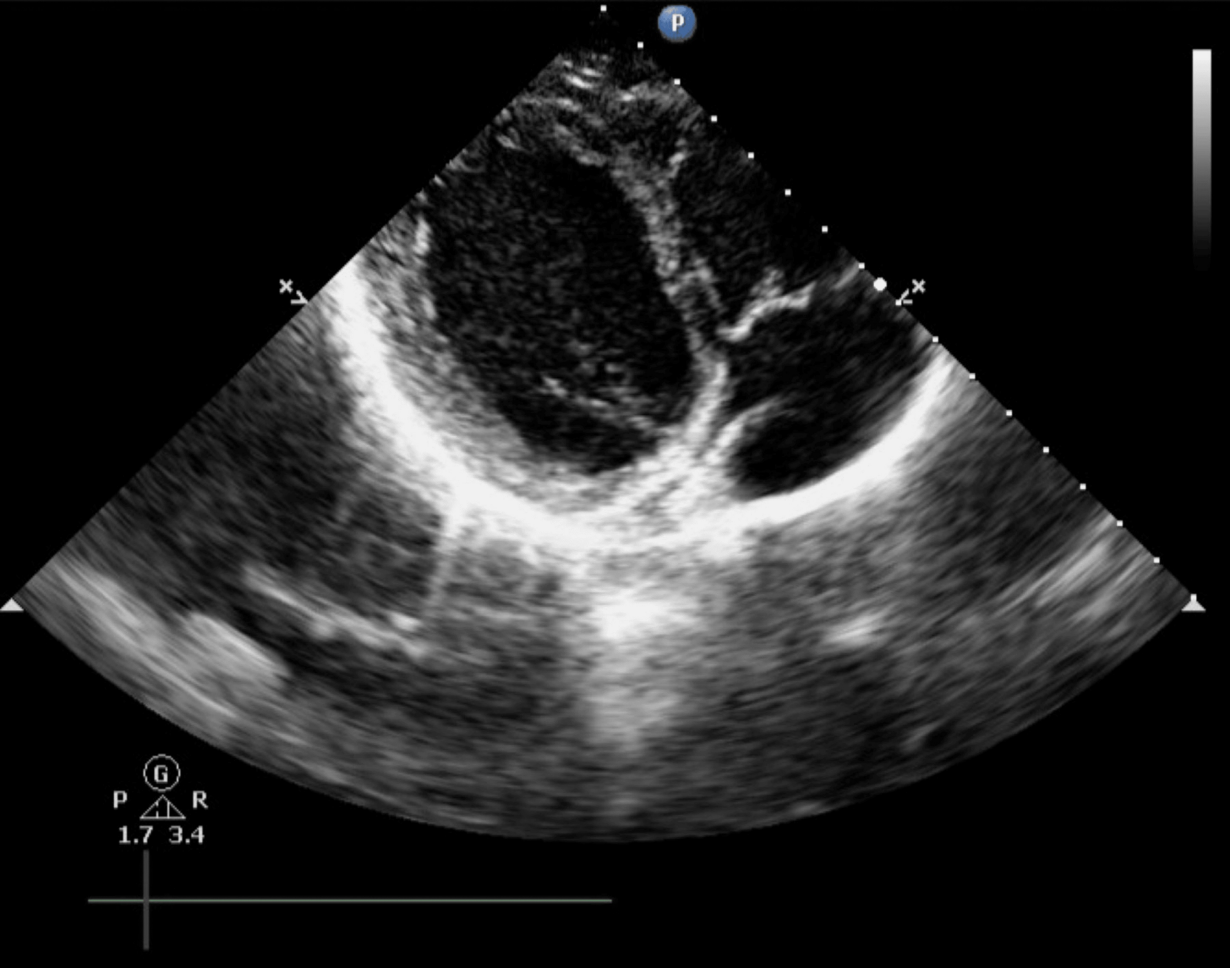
Subcostal TEE three chambers view revealing a prominent Eustachian valve within the right atrium TEE: transesophageal echocardiography
